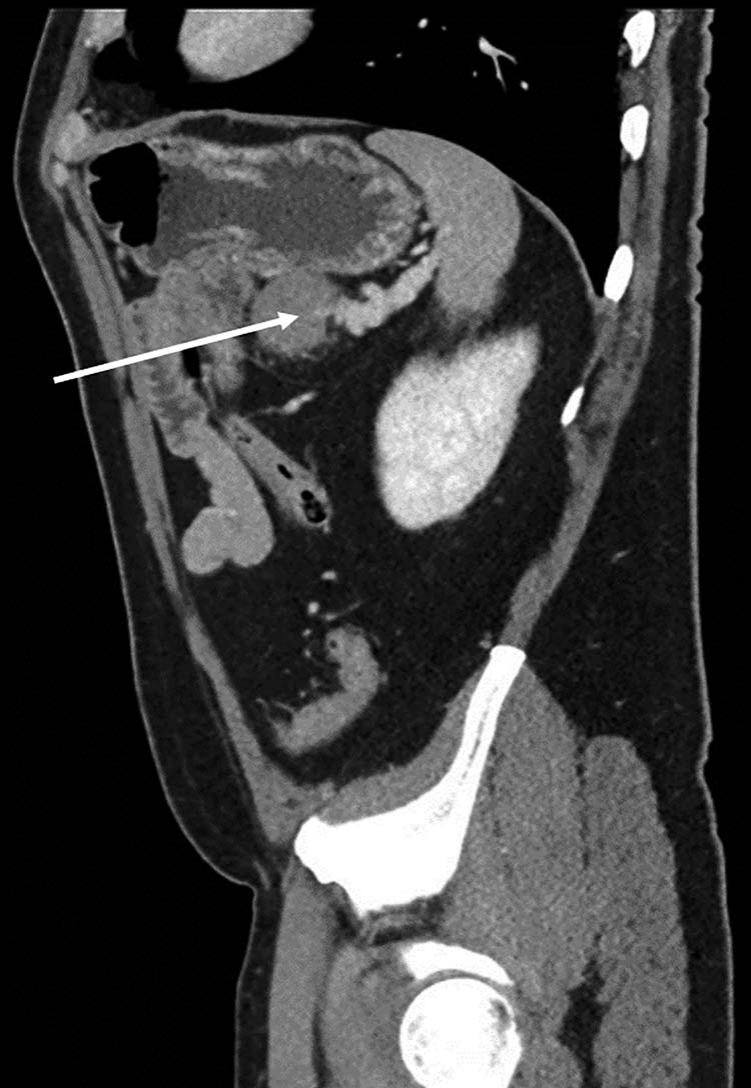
Sagital CT scan of abdomen, selected image from a pancreatic protocol, late arterial/pancreatic phase. A 3.7-cm mass, between the tail of the pancreas and the splenic hilum, causing a narrowing of the splenic vein and splenomegaly (arrow).
